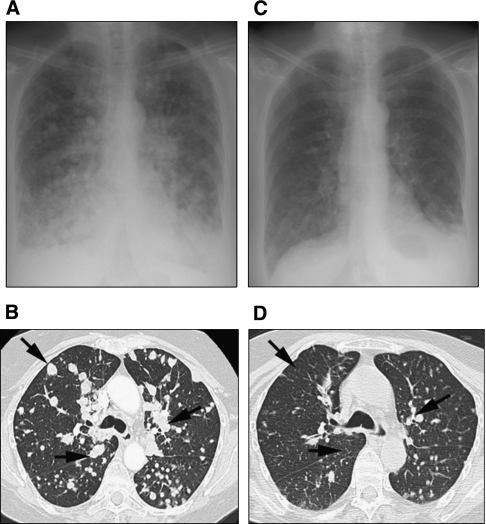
Chest X-ray and chest CT images at baseline (A, B) and then at 1 month (C, D) after treatment of a metastatic anal melanoma with sorafenib demonstrates significant reduction in the number and size of pulmonary metastases. Arrows indicate major sites of response (Case 4).
